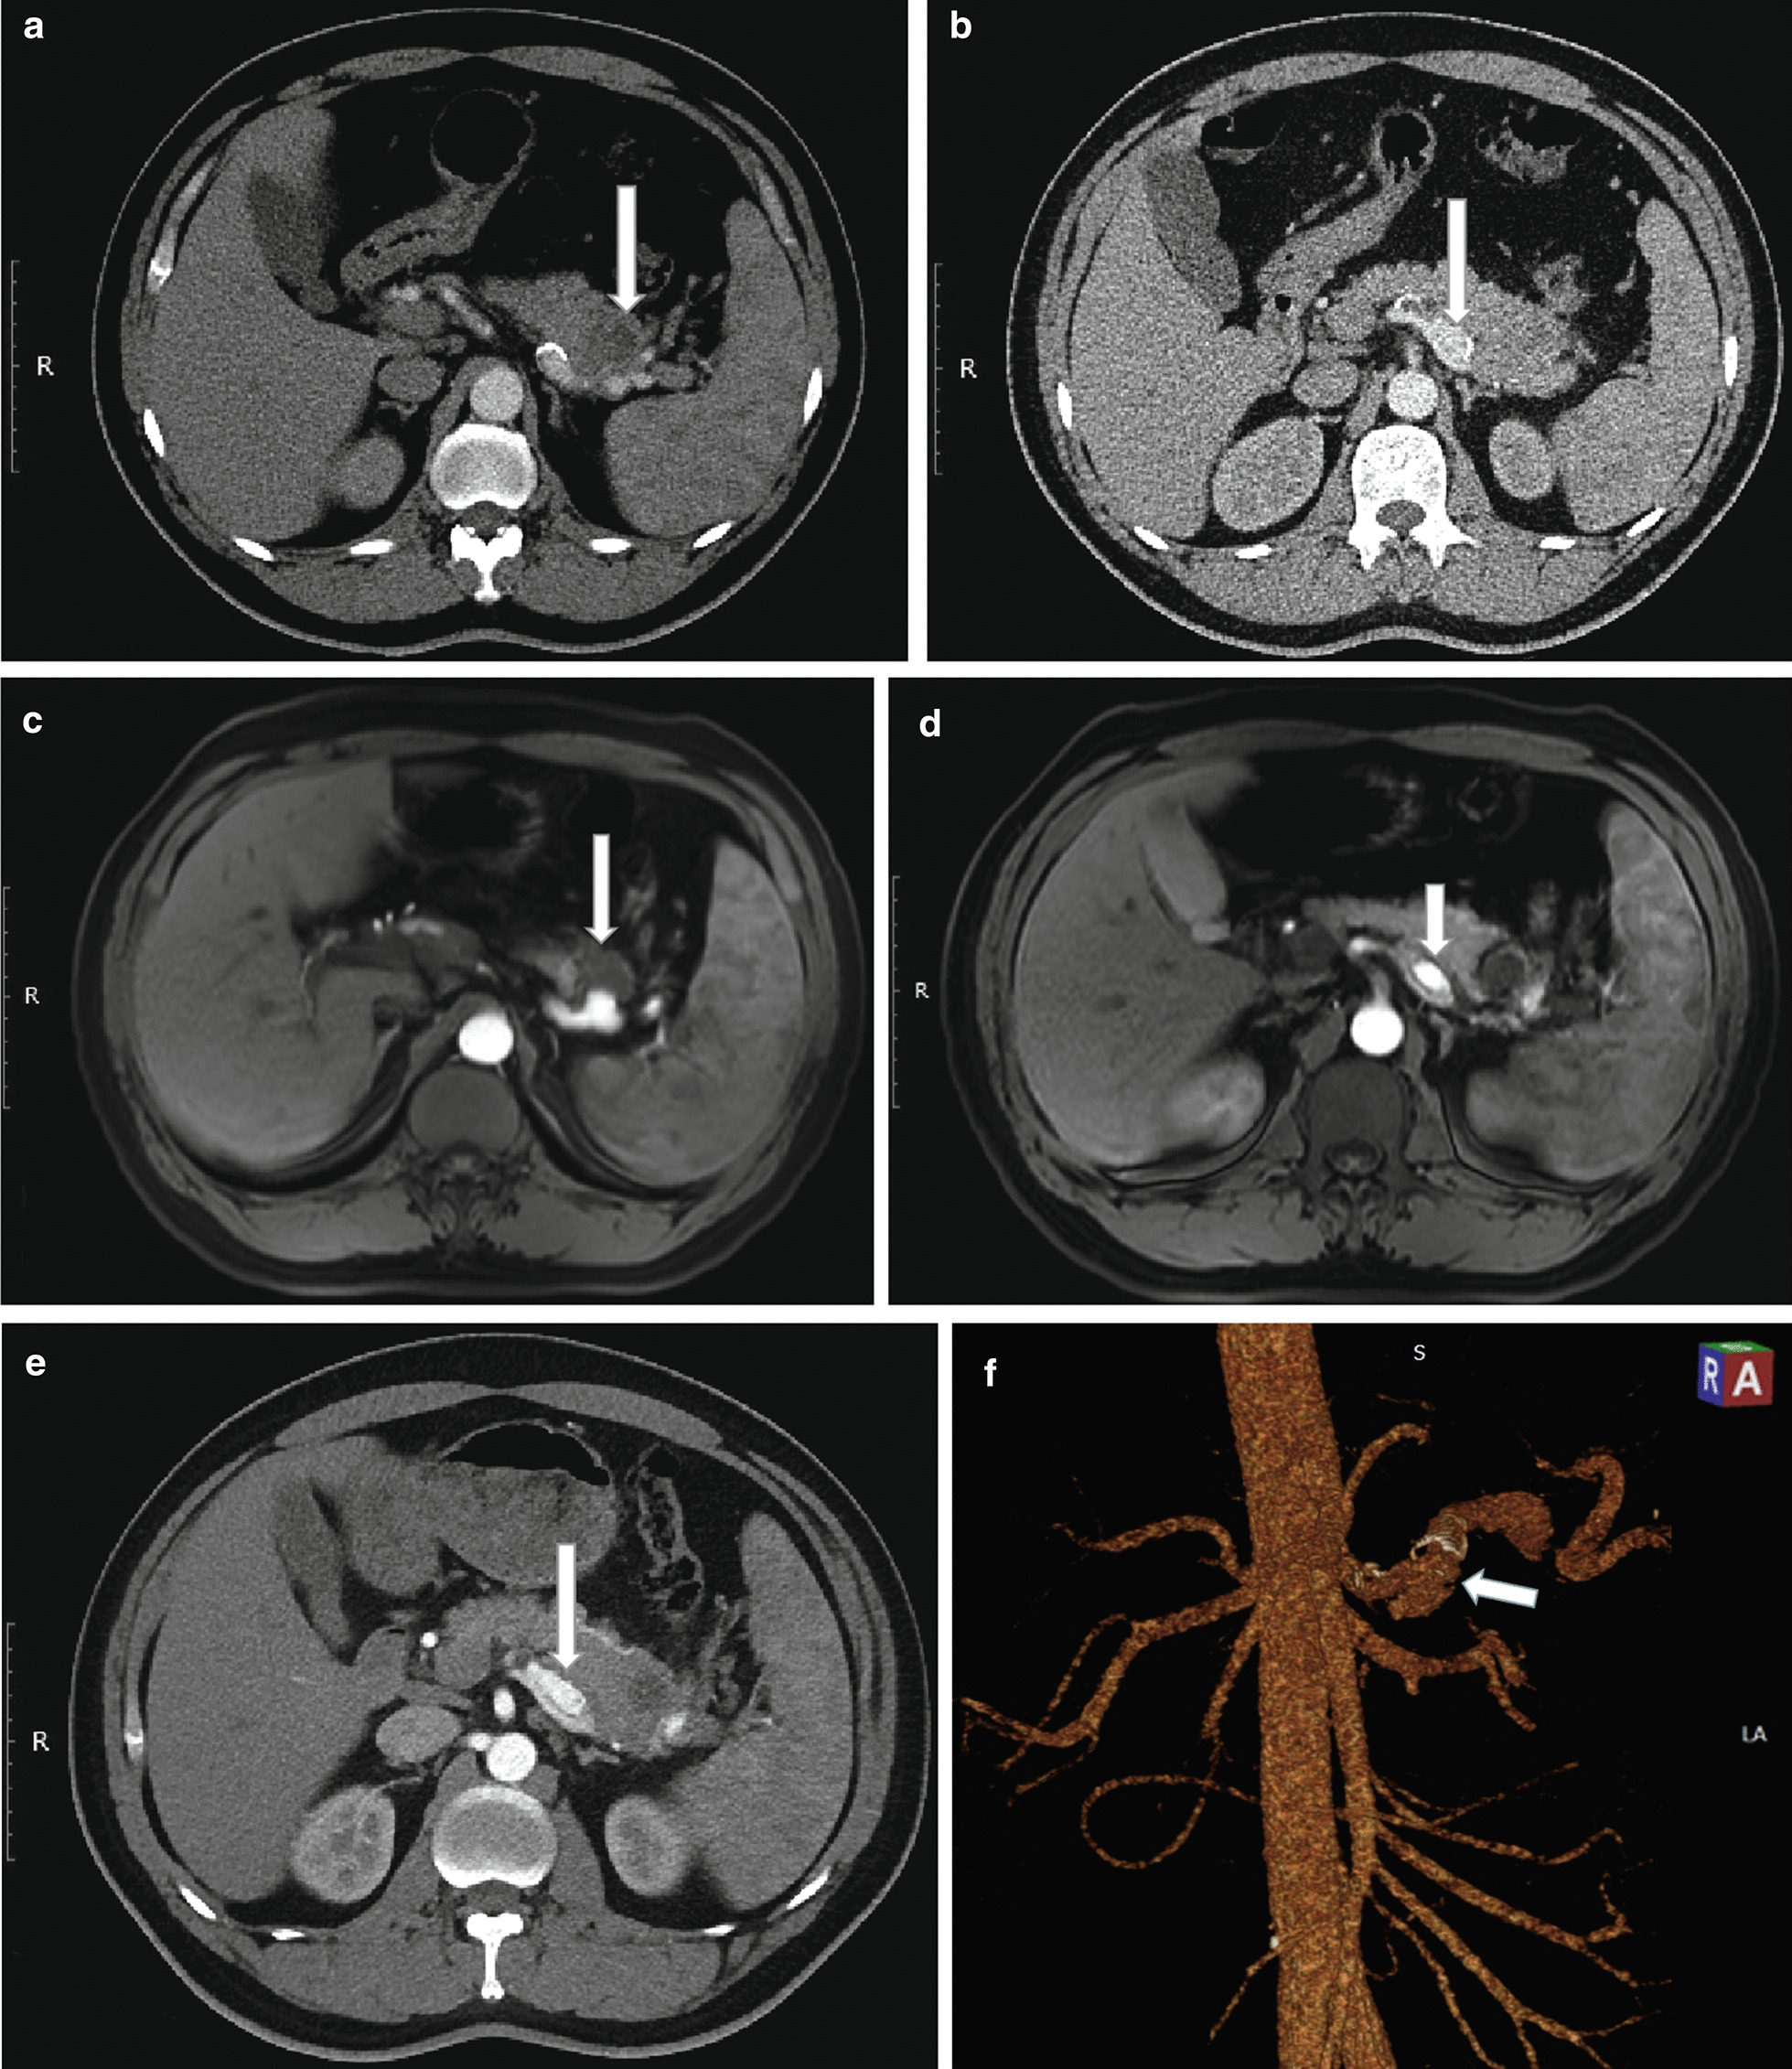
Preoperative images: a Contrast-enhanced computed tomography (CT) reveals a 3.6 × 3.4 cm^2^ intrapancreatic hypodense mass (white arrow) close to the splenic artery (SA). b CT shows dilation of the calcified SA (white arrow). c Magnetic resonance angiography shows a hematoma (white arrow) close to the protruding SA. d The proximal SA is divided into two lumens. e, f CT Angiography confirms isolated splenic artery dissection (SAD) with a typical “double-lumen” sign (white arrows). The SA was partially thrombosed in the proximal segment
